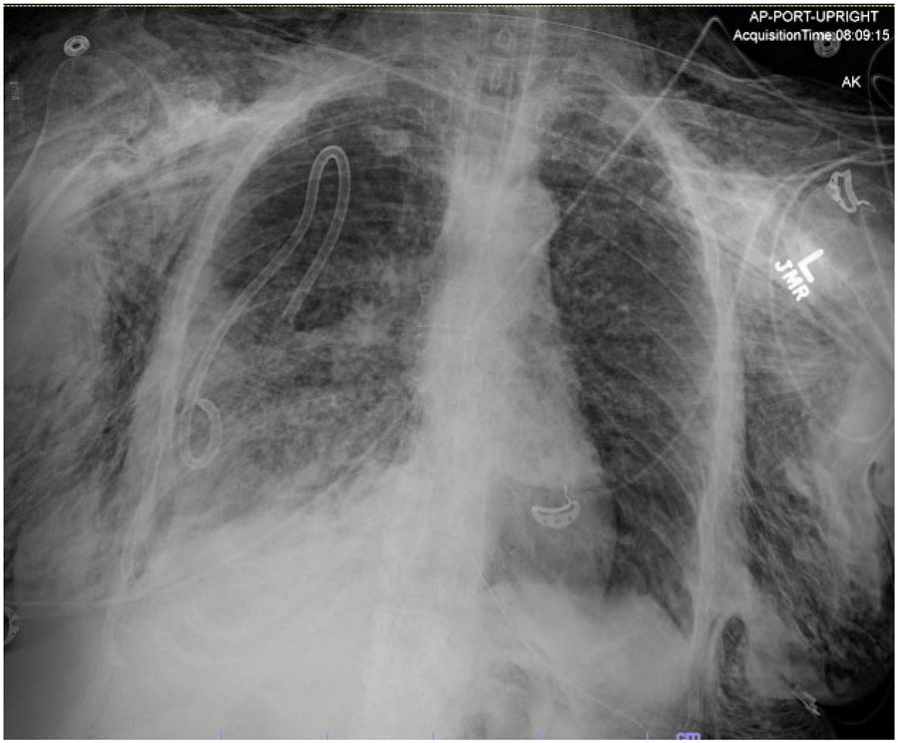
Post-operative chest radiograph showing increased diffuse subcutaneous emphysema in chest, lower neck and upper left abdominal wall. Previously demonstrated pneumoperitoneum is not visualized on the current film.
